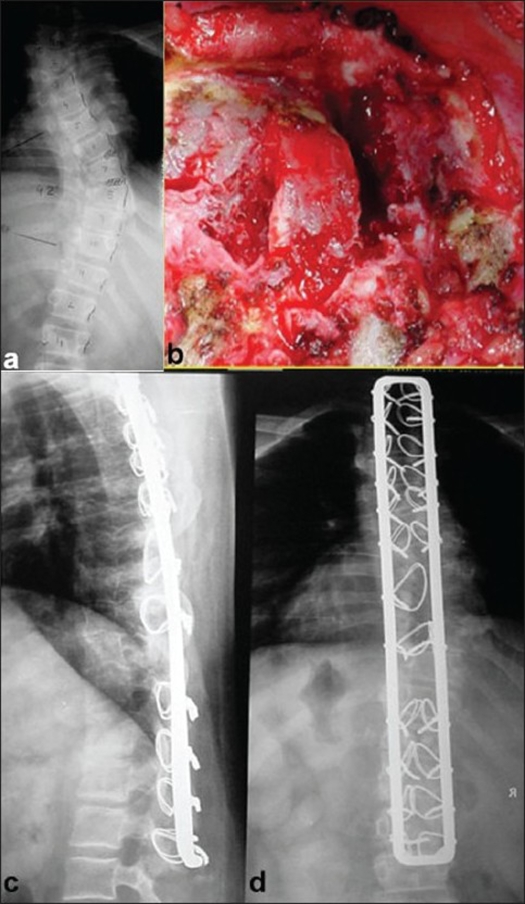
X-ray (Anteroposterior) of dorsolumbar spine (a) shows congenital scoliosis, (b) shows anterior epiphsiodesis. Postoperative X-ray lateral, (c) and Anteroposterior, (d) view of the same patient shows good correction and balance in sagittal and coronal planes
